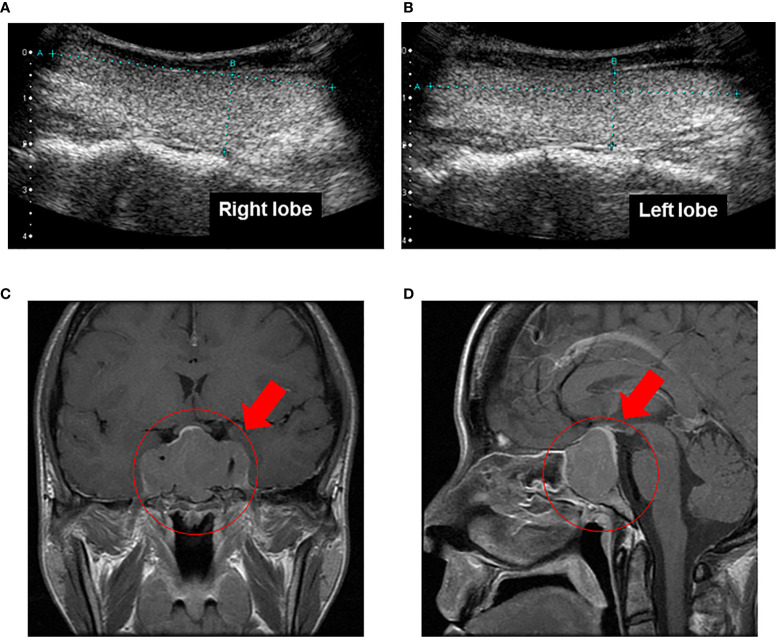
(A, B) In thyroid echography, both lobes of the thyroid gland were swelling although increase of blood flow was not observed. (C, D). In brain magnetic resonance imaging, there was a giant tumor (51 × 34 × 22 mm) around pituitary fossa, pressuring on optic chiasm from the middle. Bilateral internal carotid arteries were surrounded by the tumor, and infiltration into the cavernous sinus was observed.
